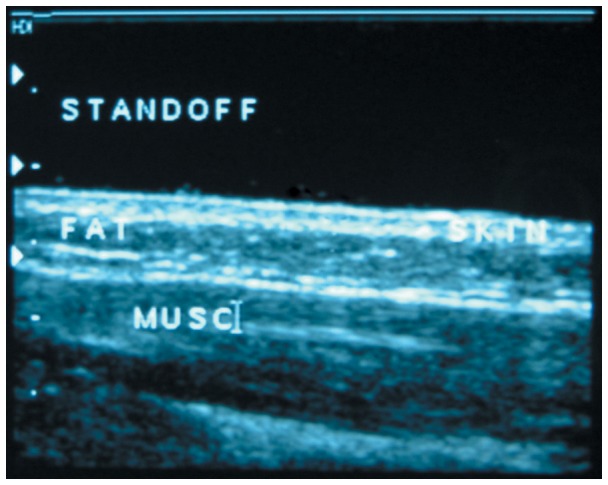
Ultrasound image showing a cross-sectional view of the standoff, dermis (skin), subcutis (fat), and muscle tissue (musc).
